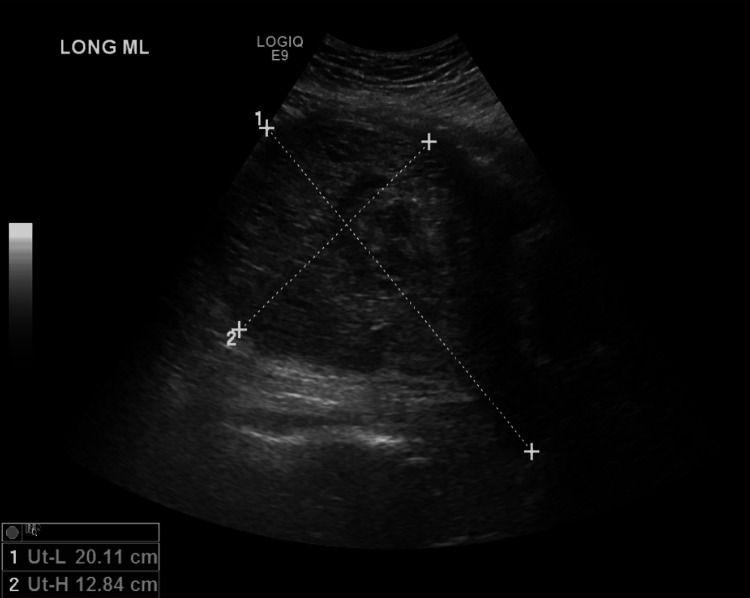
Transabdominal ultrasound imaging demonstrating enlarged uterus measuring 20.11x12.84x16.9 cm. Endometrial cavity with pathognomonic "snowstorm" appearance, suggesting the presence of many hydropic villi.
